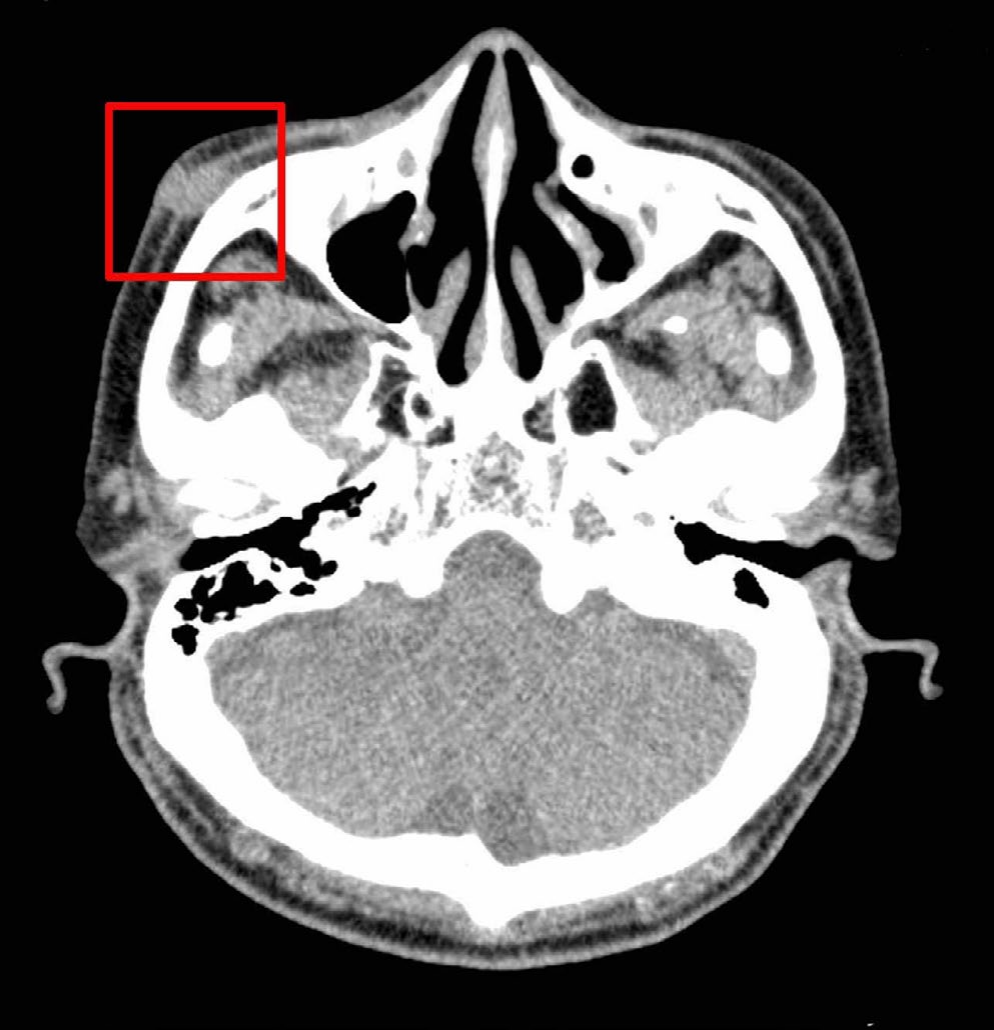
Plain CT image of the cheek. A mass in contact with the zygomatic bone can be observed in the framed area
